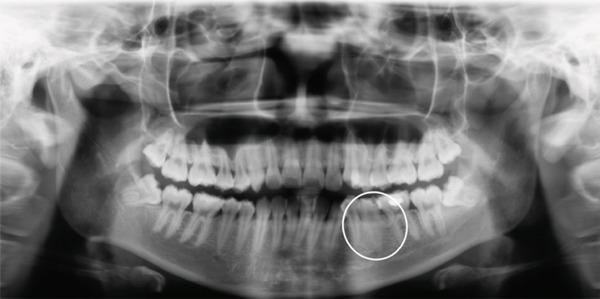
Panoramic radiography at the first visit.
